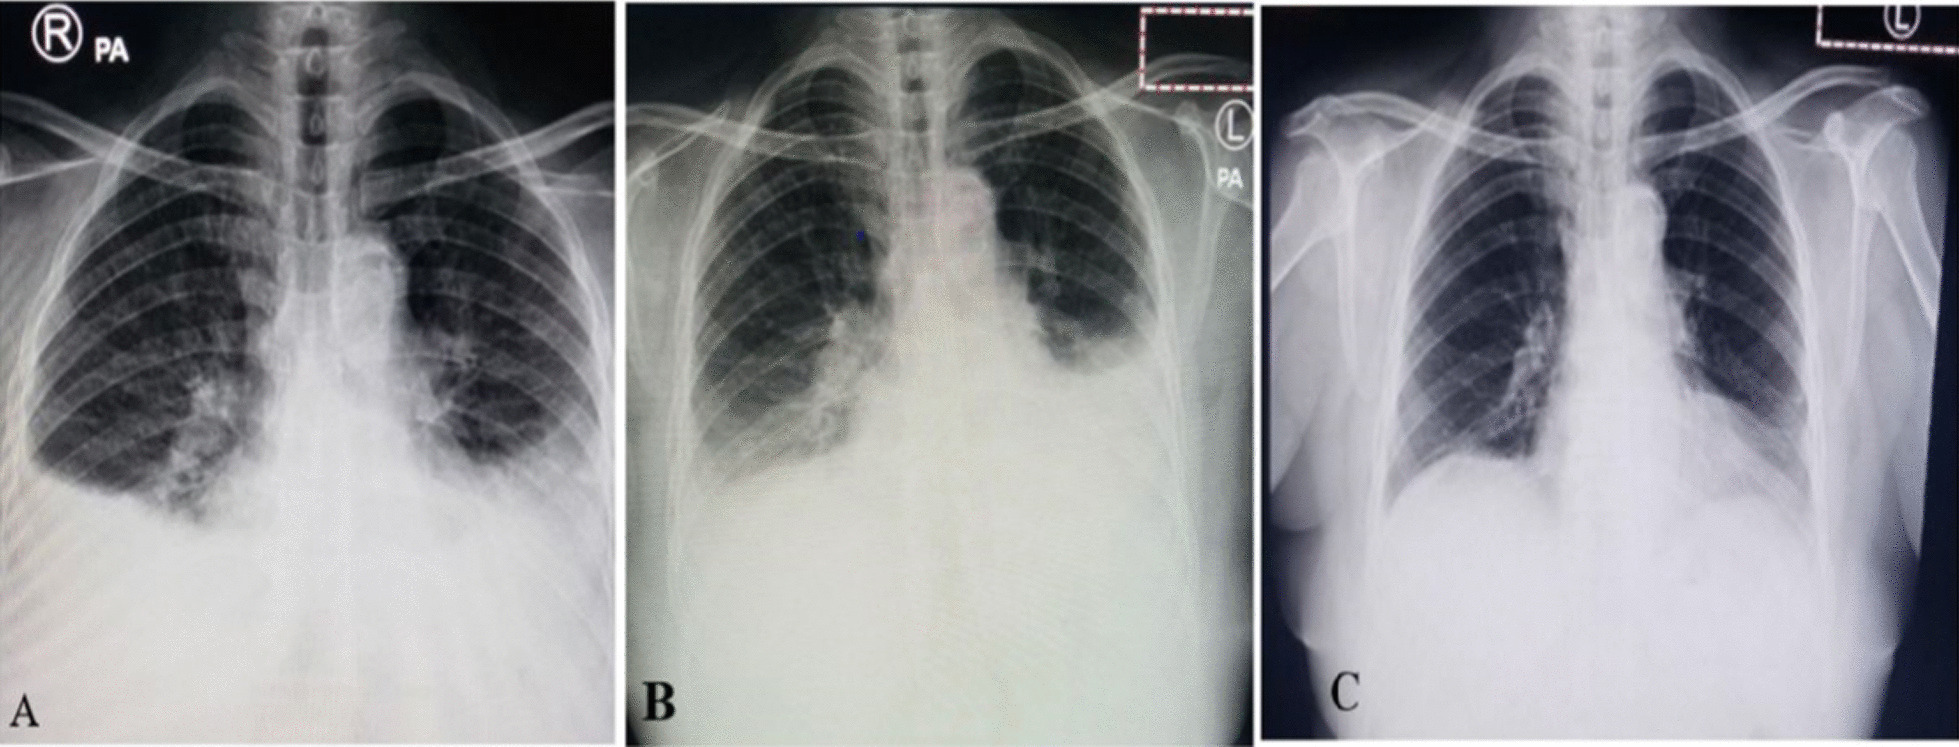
Serial chest X-ray images. A Symmetrical bilateral pleural effusion; B bilateral pleural effusion greater on the left side; C significant improvement, except for left basal haziness
